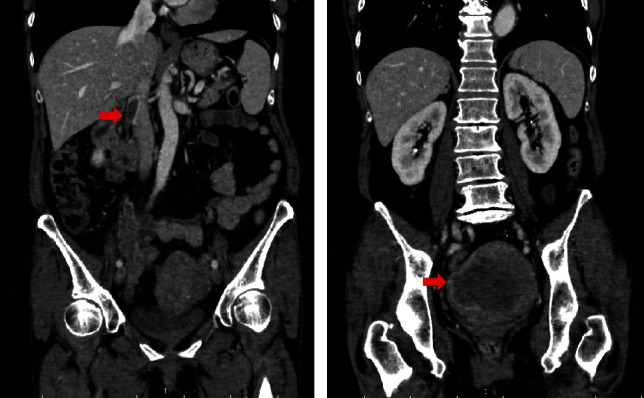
Computed tomography on admission. Intraluminal filling defect from the right ovarian vein to the inferior vena cava (arrow) (a) and a large uterine mass (arrow) (b).
